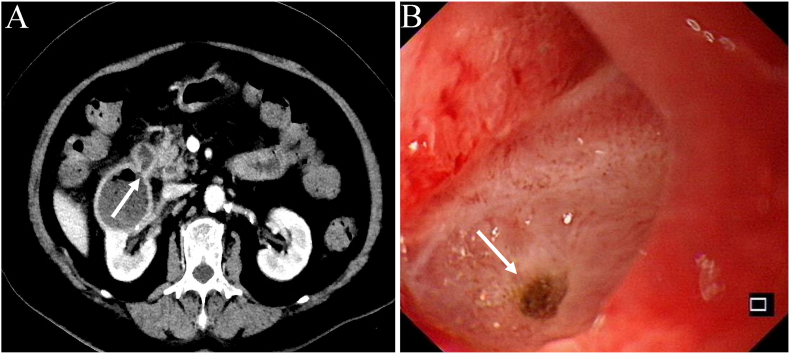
(A) An axial contrast-enhanced CT scan revealed the presence of an air-fluid level within the right renal cyst, characterized by a fistulous tract forming a connection with the duodenum (white arrows). (B) Upper gastrointestinal endoscopy demonstrated a circular ulcer located within the duodenal bulb (white arrows).
